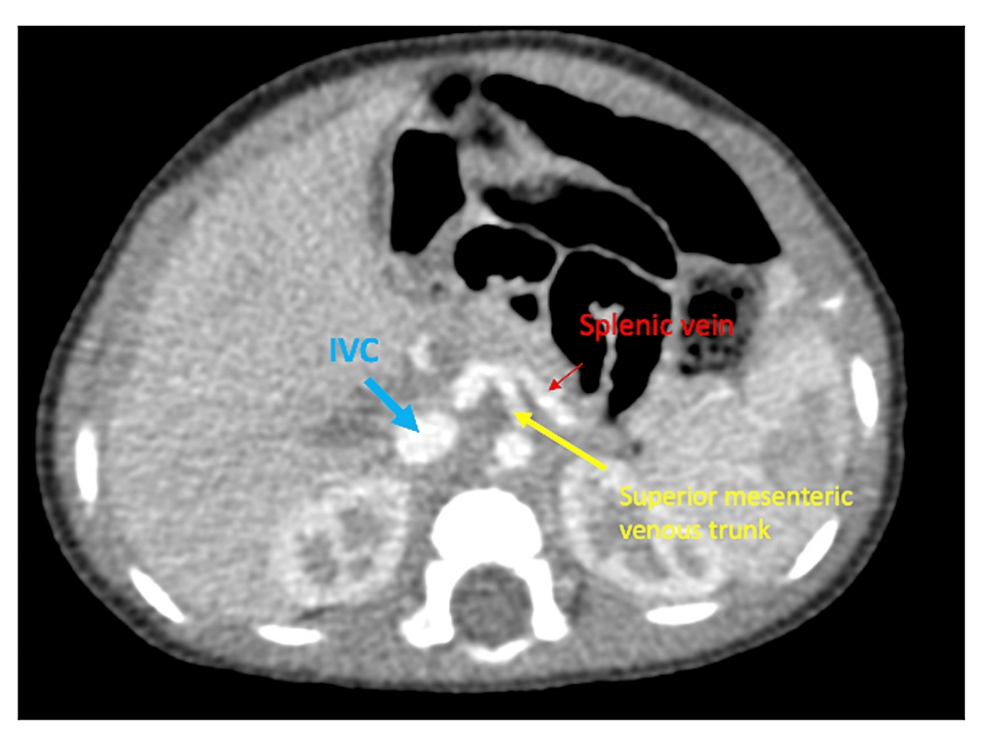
CT scan of the abdomen (axial view): confluence of the superior mesenteric venous trunk and the splenic vein into the IVC. CT: computed tomography; IVC: inferior vena cava
